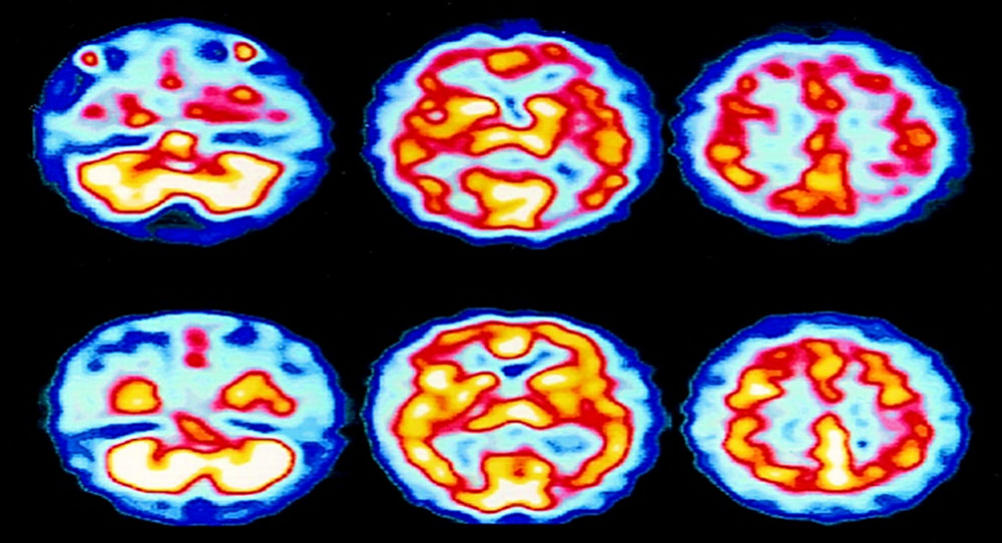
Brain SPECT images [24].
